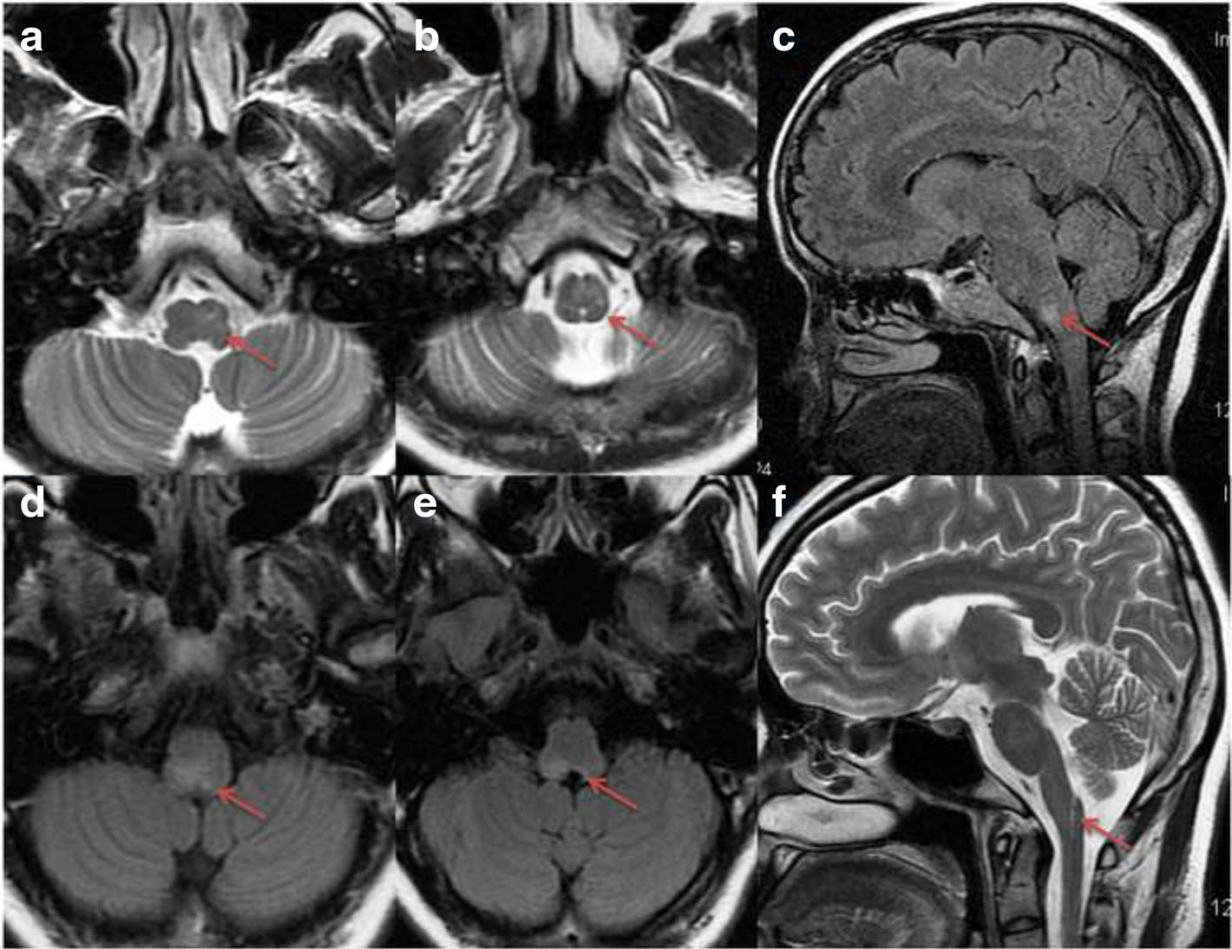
Axial T2-weighted (a and b; *arrow*) and sagittal T2 FLAIR (c; *arrow*) MRI show lesions occur in the medulla oblongata. Axial T2 FLAIR (d and e; *arrow*) MRI show pericanal lesions occur in the medulla oblongata. Linear faint Sagittal-T2-weighted high intensity signals are shown in the medulla oblongata (f; *arrow*)
